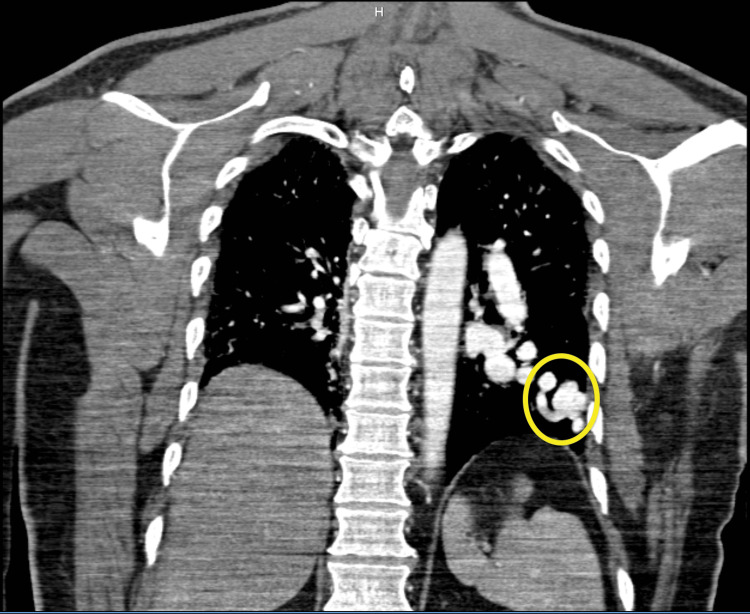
CT pulmonary angiogram (coronal view) showing a left lower lobe PAVM with perfusion defect (yellow circle). PAVM: pulmonary arteriovenous malformation
